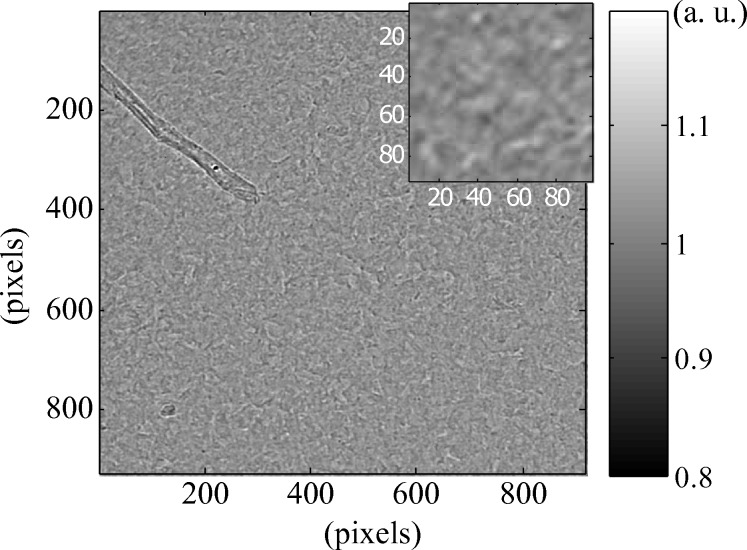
Effect of the semi-transparent mirror and first detector scintillator on the X-ray beam as seen by the second detector.
